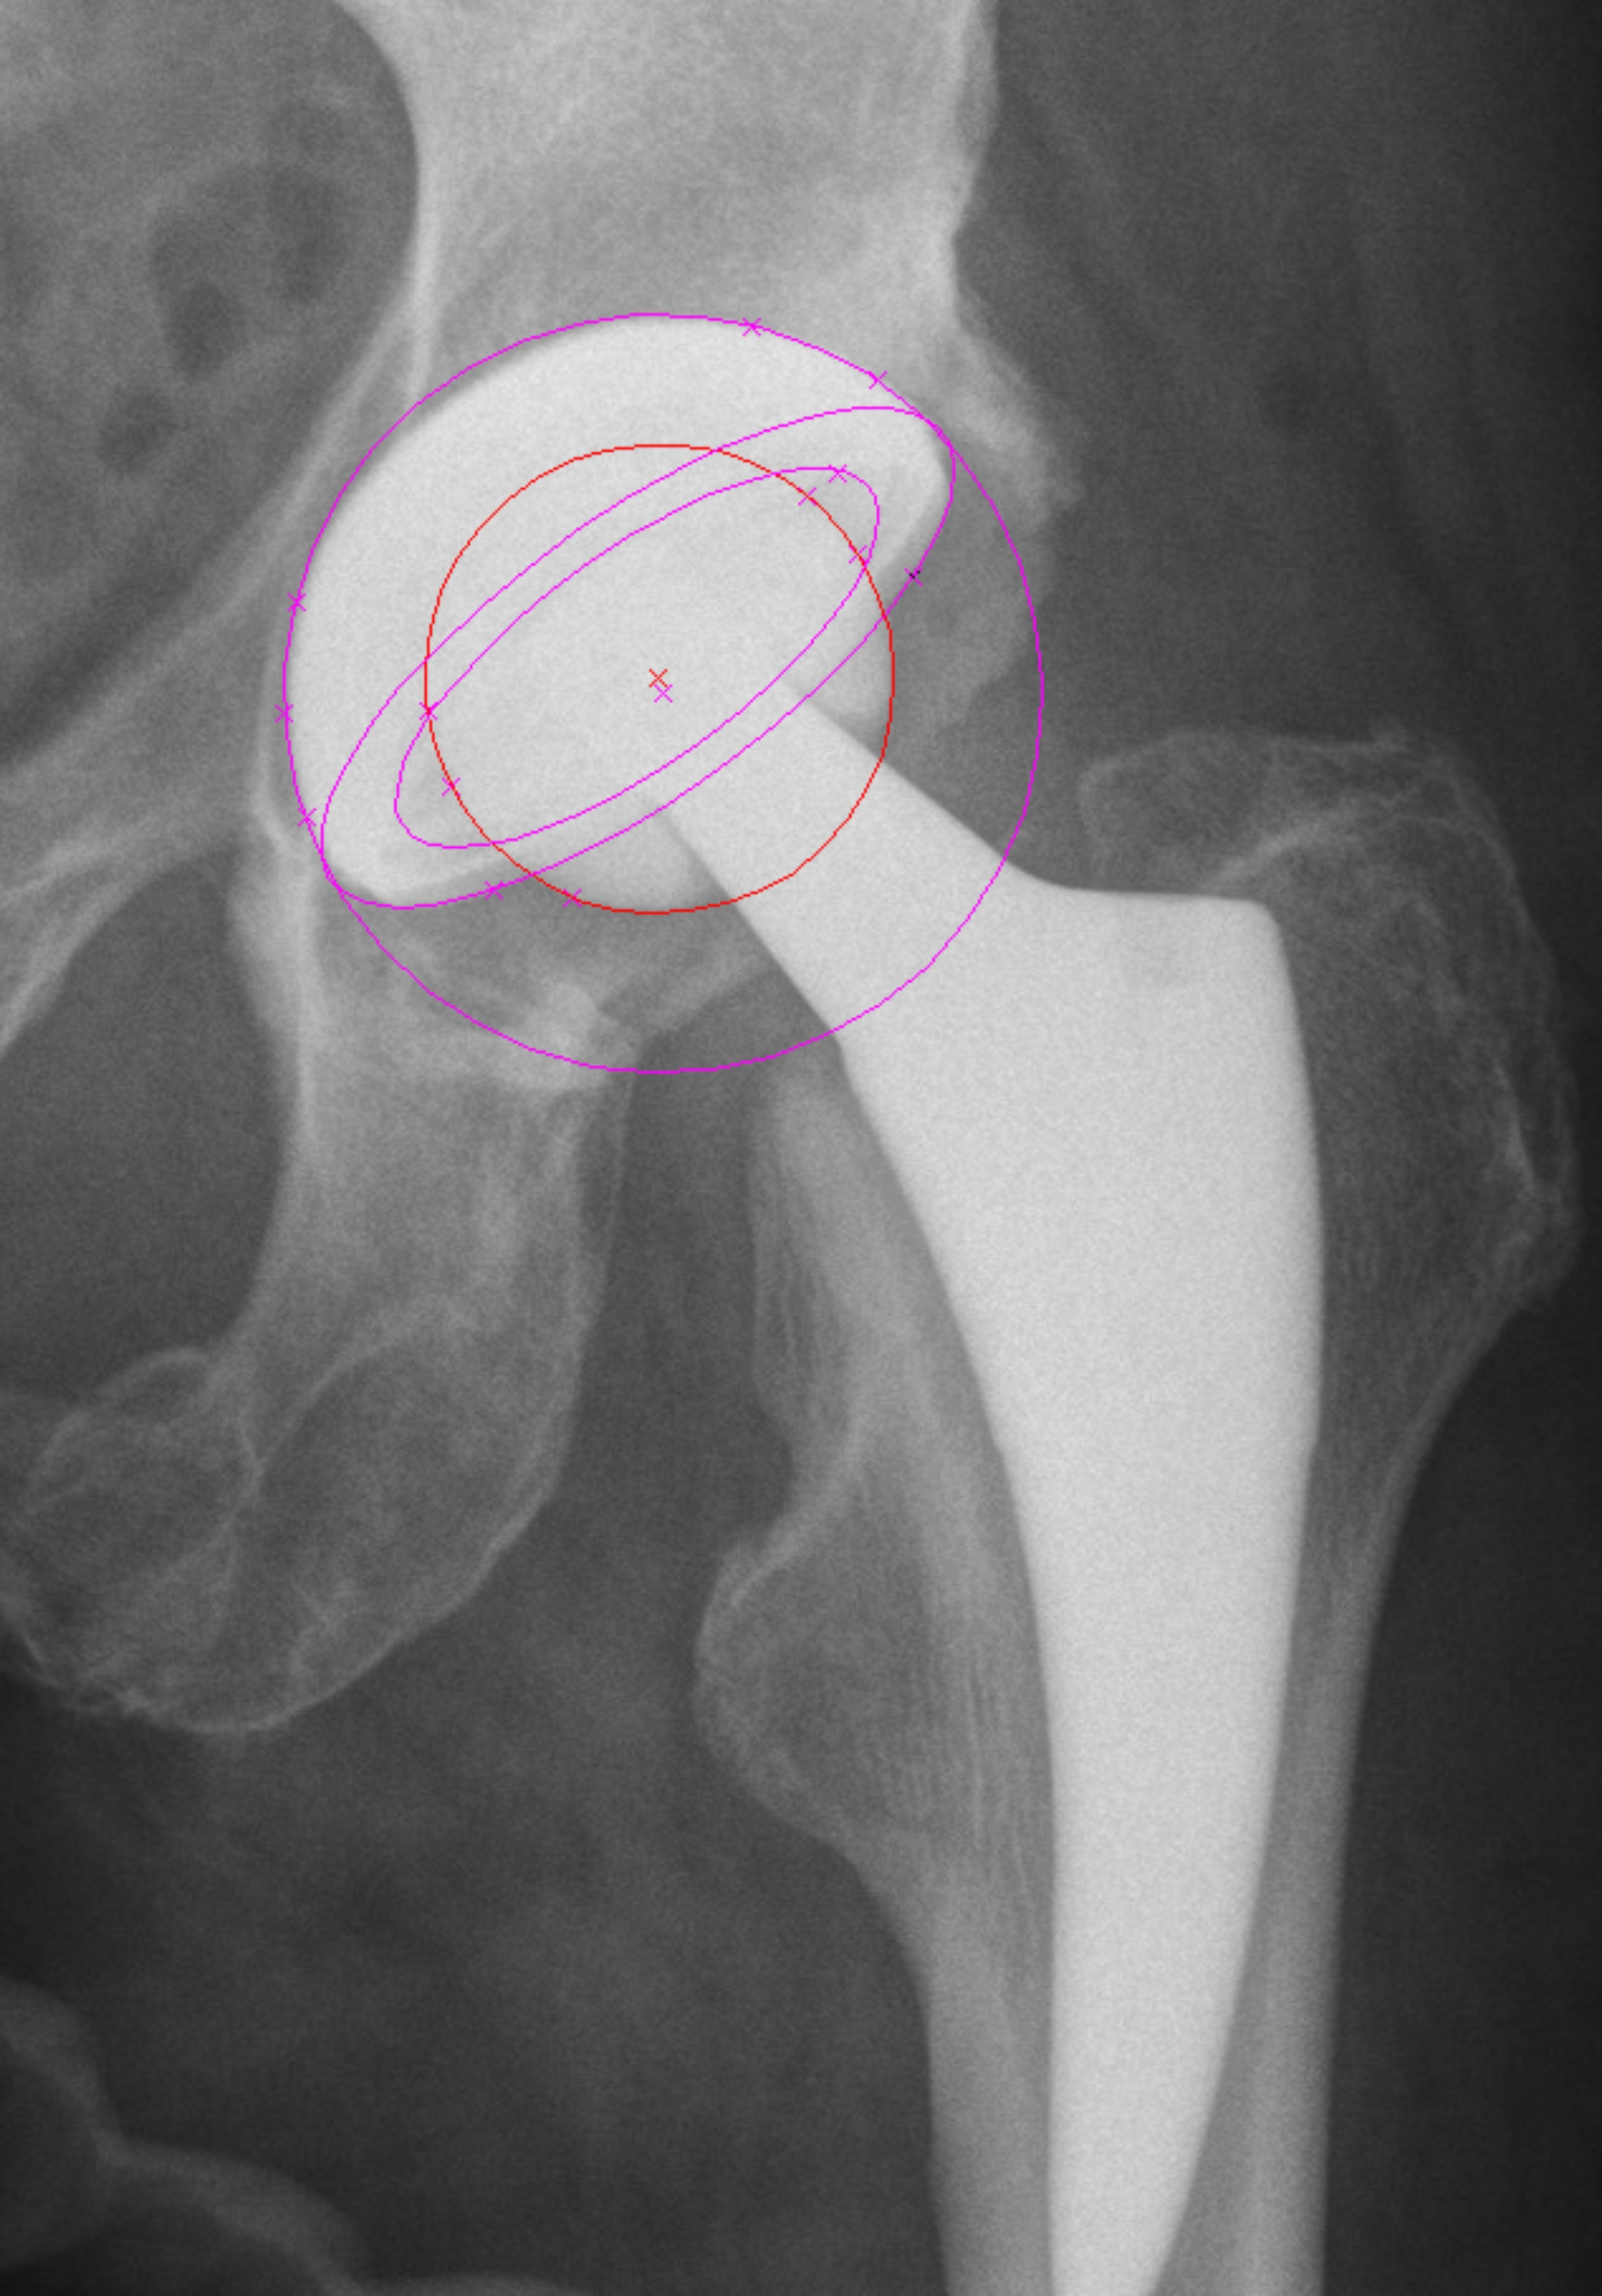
Wear measurements using PolyWare® shown in ap-Radiographs
